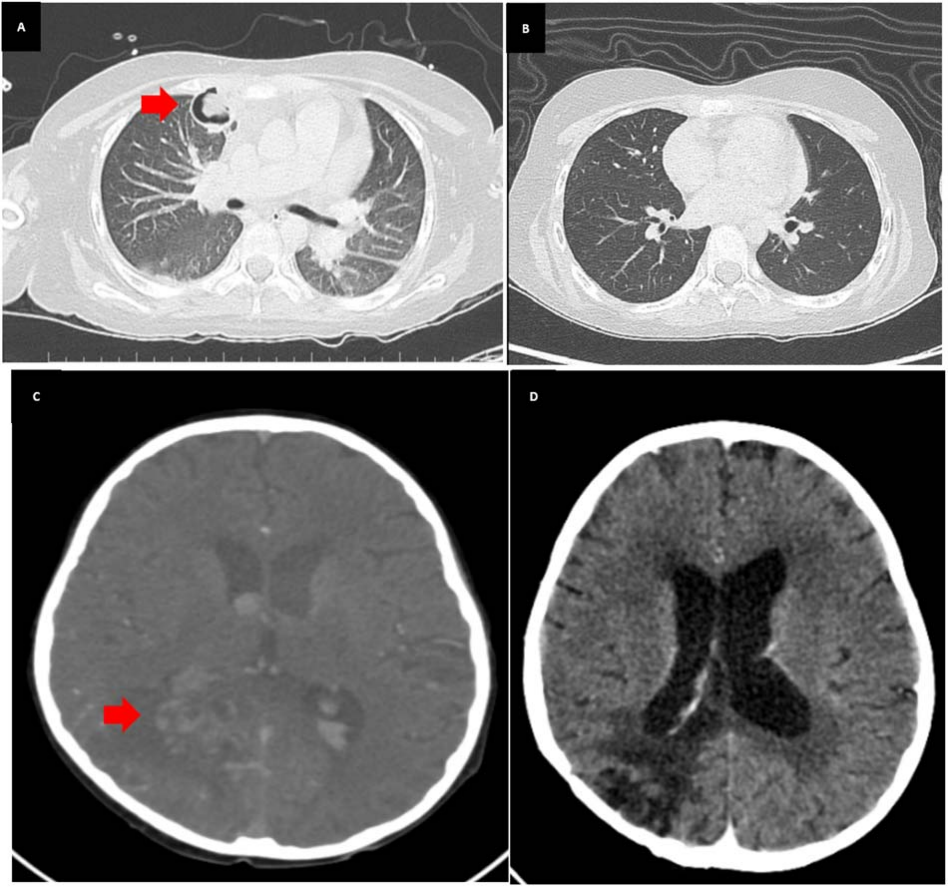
CT chest and CT brain before and after treatment in this patient. (A) Contrast-enhanced CT of the chest detected an air crescent sign at the anterior segment of the right upper lobe of the lungs. (B) Absence of air crescent sign after treatment. (C) CT angiography of the brain revealed an outpouching lesion at the right posterior cerebral arteries. (D) After treatment and follow-up, the ruptured aneurysm and intraventricular hemorrhage improved.
